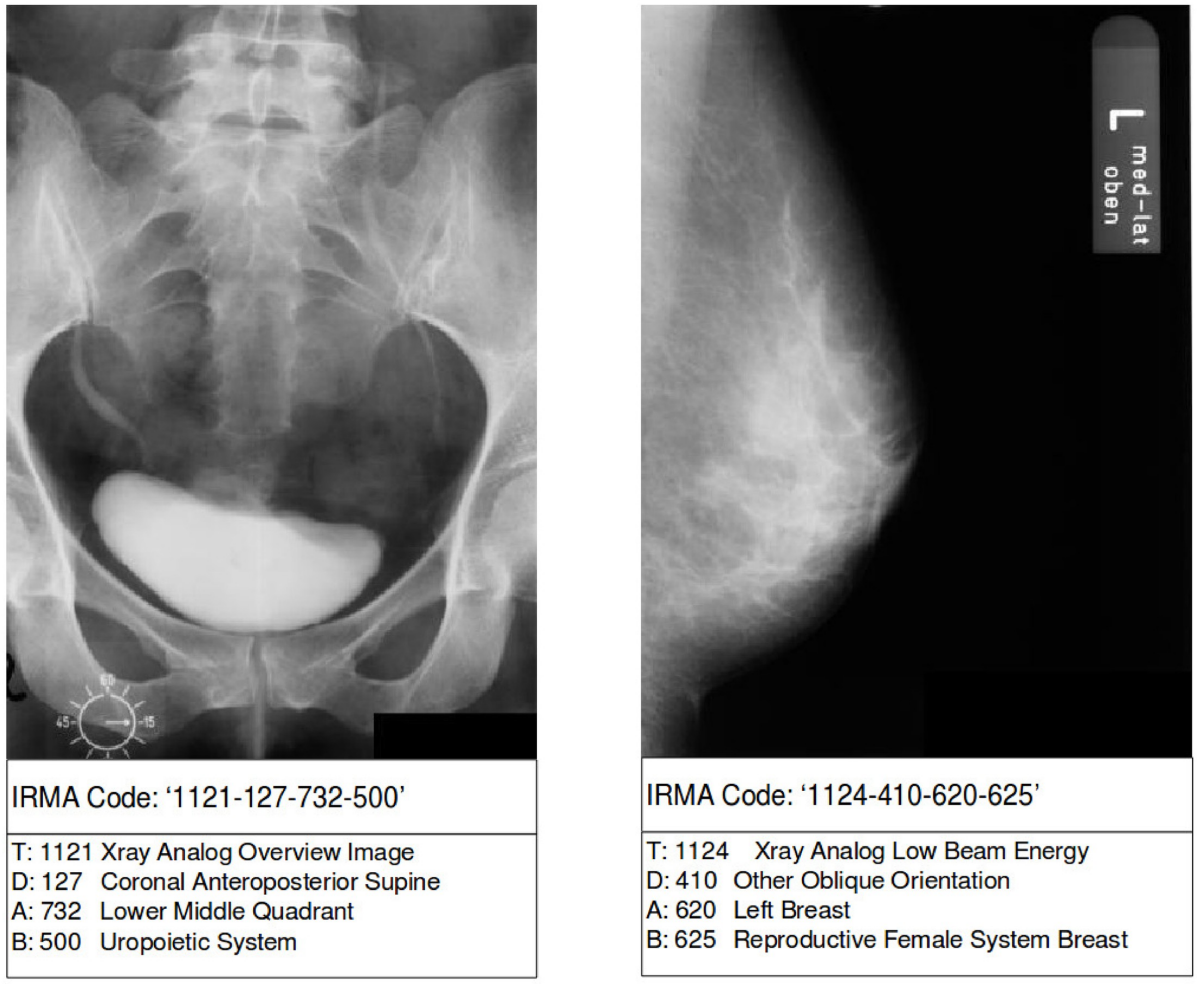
Example of two grayscale radiographs annotated with the 13-digit classification code. Both images were randomly chosen from the ImageCLEF 2009 Medical Annotation Task Training Set. Republished from [21] under a CC BY license, with permission from [RWTH Aachen], original copyright [2009].
